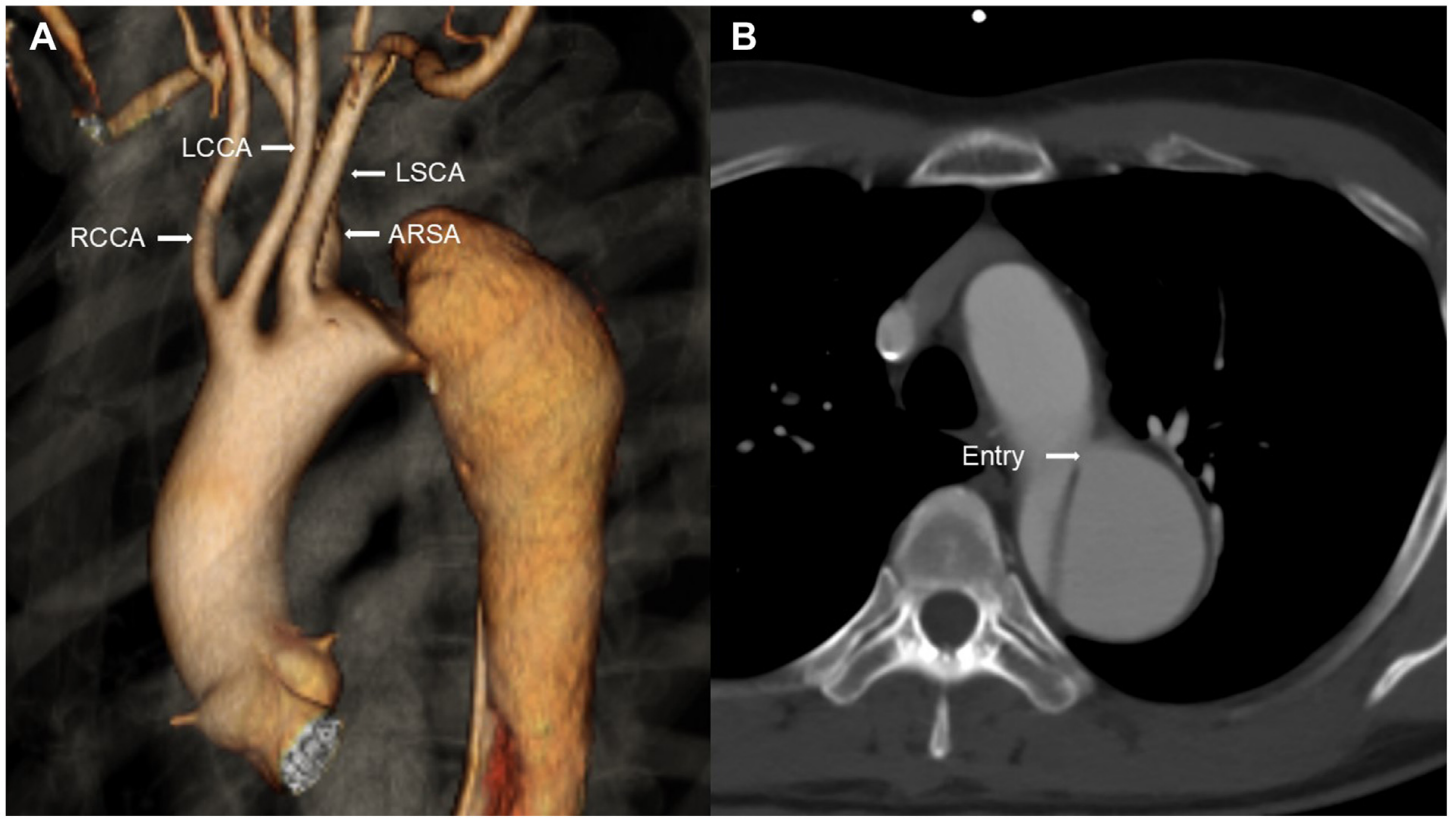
Preoperative contrast-enhanced computed tomography (CT) images. (A and B) CT imaging with contrast revealed Stanford type B aortic dissection (TBAD) with an entry tear in zone 3 with an aberrant right subclavian artery (ARSA). CT scan showed that the entry of the aortic dissection was located below the ARSA. The sequence of the cervical branches was as follows: a common origin of the right common carotid artery (RCCA) and left common carotid artery (LCCA); left subclavian artery (LSCA); and ARSA. The ARSA originated from the aortic arch.
